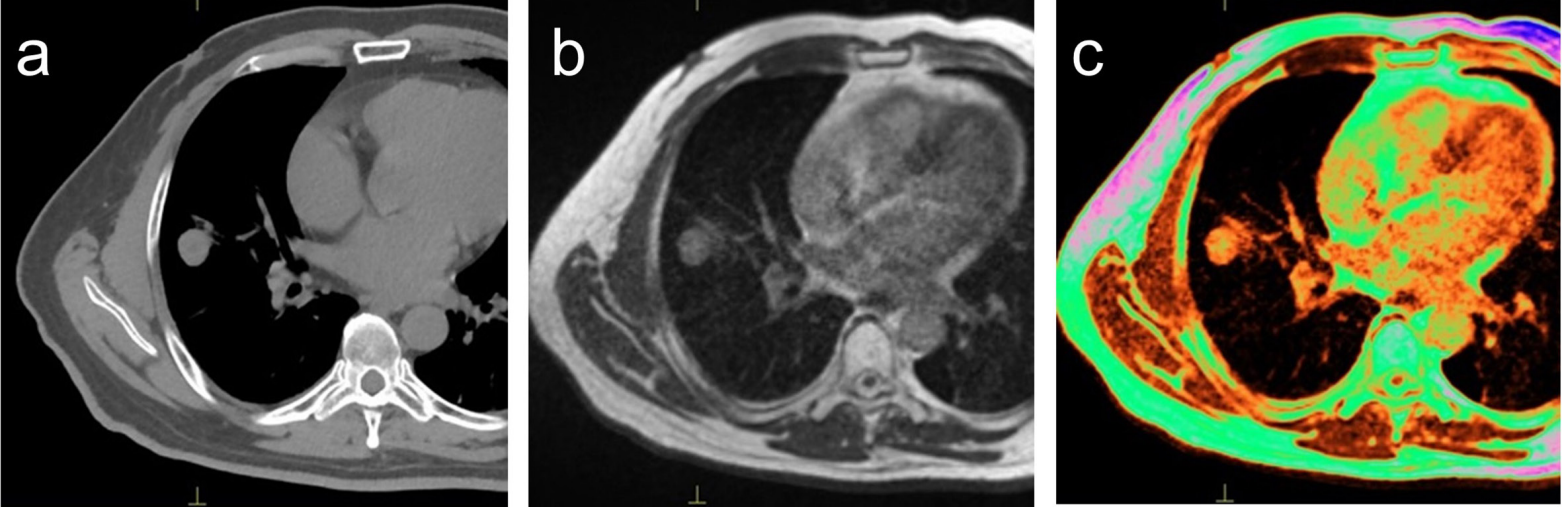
Measurement of GTV. A rigid fusion of both MRI (a) and CT (b) was performed prior to contouring. GTV based on CT (GTV-CT) was defined on the planning CT with a window level of 30–50 HU and window width of 350–500 HU. GTV based on MRI (GTV-MRI) was defined using fusion image (c) in order to avoid mistakenly containing normal tissues such as blood vessels. GTV, gross target volume.
